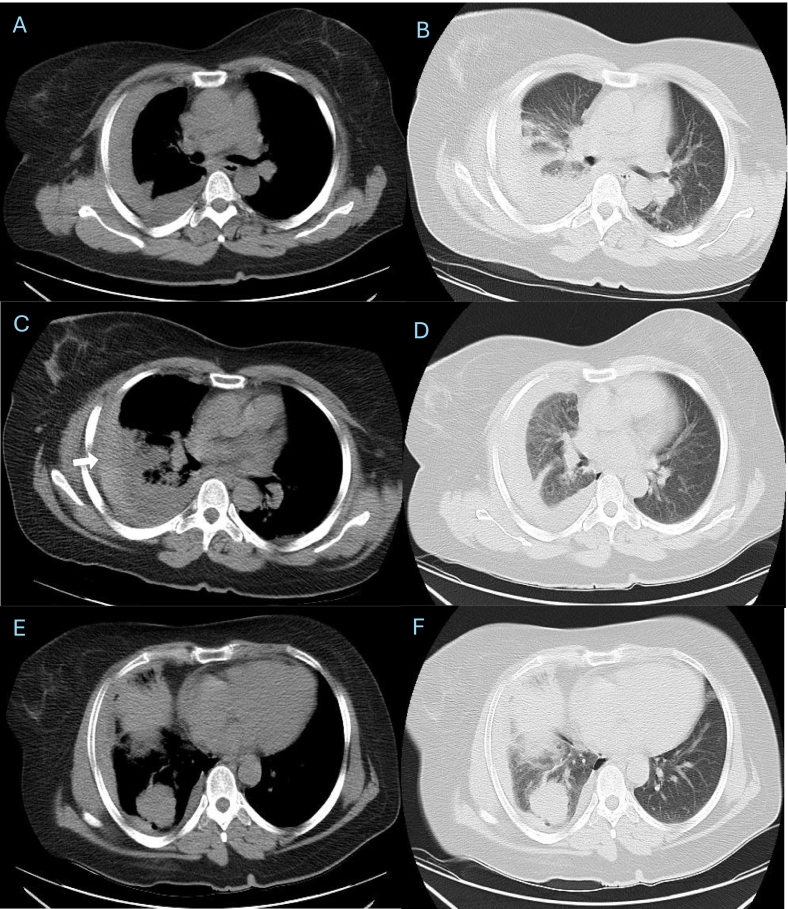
Axial CT images in the supine position obtained 30 min post-biopsy showing increased right pleural fluid. The newly developed high-density component indicates early hemorrhagic transformation (white arrow).
